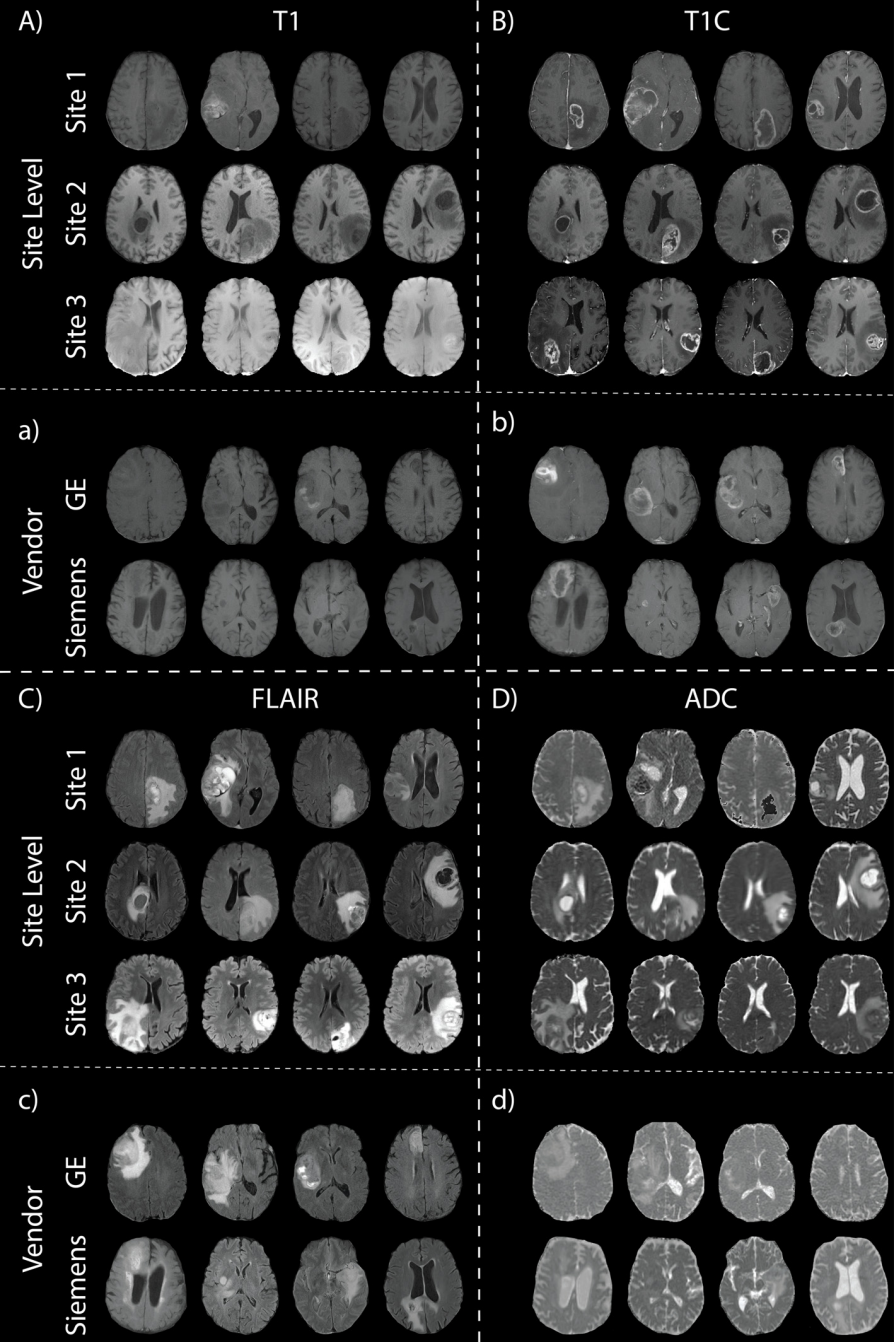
Glioblastoma imaging. T1 (A), T1C (B), FLAIR (C), and ADC (D) images for four patients across the three data sites. Additionally, examples of images scanned on the GE and Siemens scanners are shown (a, b, c, d, with respect to acquisition).
